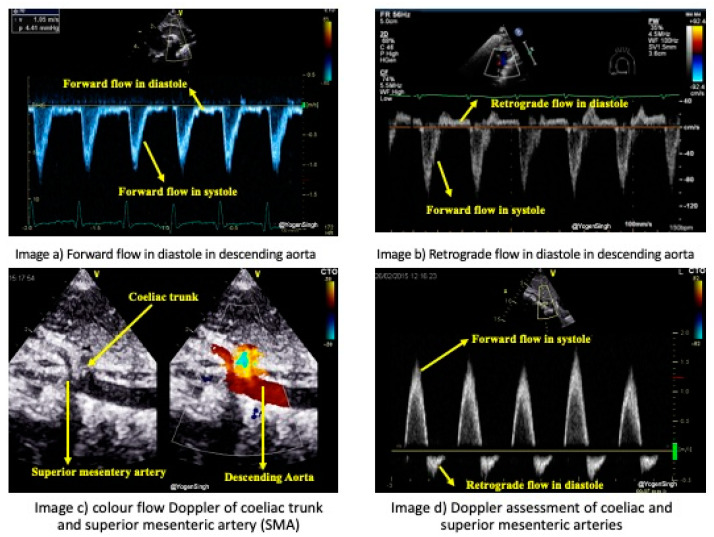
Doppler assessment of blood flow in the descending aorta post-ductally, in the coeliac trunk, and the superior mesenteric artery. (a) shows forward blood flow during systole and diastole in the descending aorta from the suprasternal arch view. (b) shows retrograde blood flow during diastole in the descending aorta, indicating ‘ductal steal’ in the presence of an hsPDA. (c) shows the coeliac and superior mesenteric arteries from the subcostal longitudinal color compare view, and (d) shows retrograde blood flow during diastole in the coeliac and superior mesenteric arteries, indicating ‘ductal steal’ similar to flow in the descending aorta.
